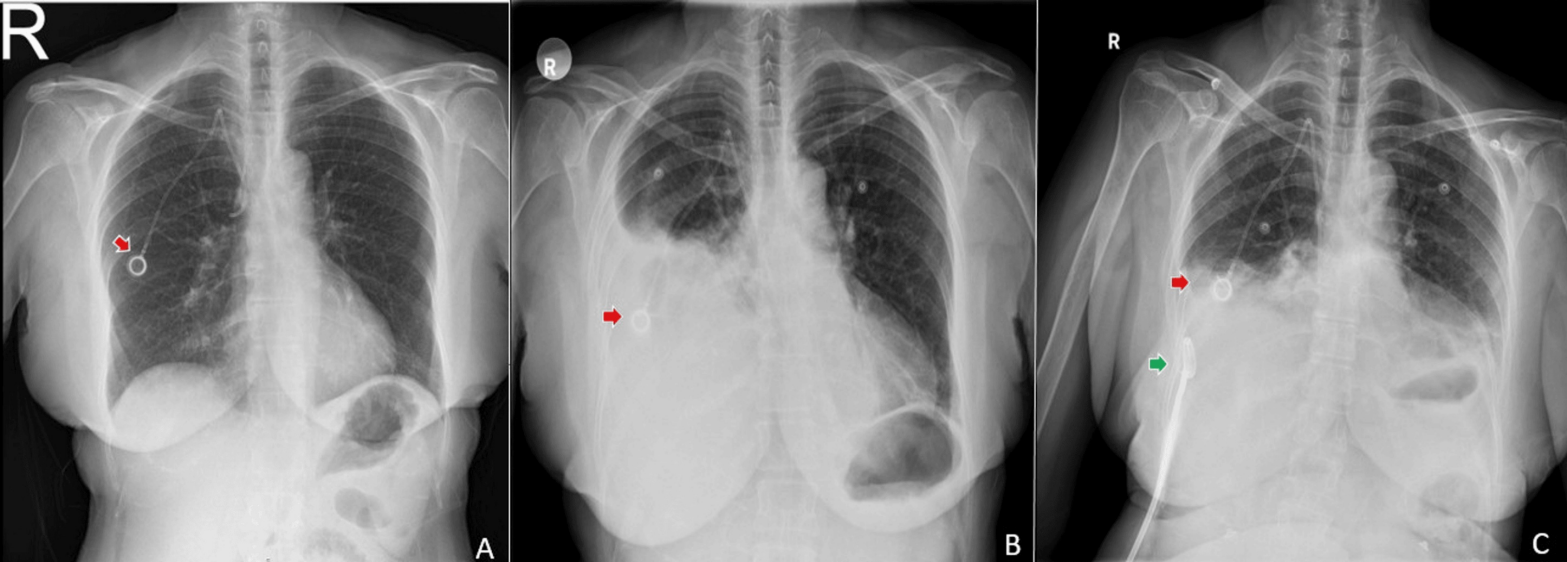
Preliminary chest radiographs of the patient. The port catheter is indicated by the red arrow, and the pigtail catheter is indicated by the green arrow. A: Control X-ray after port placement; B: Initial evaluation in the emergency room, showing right-sided pleural effusion; C: Control X-ray after pigtail catheter placement
